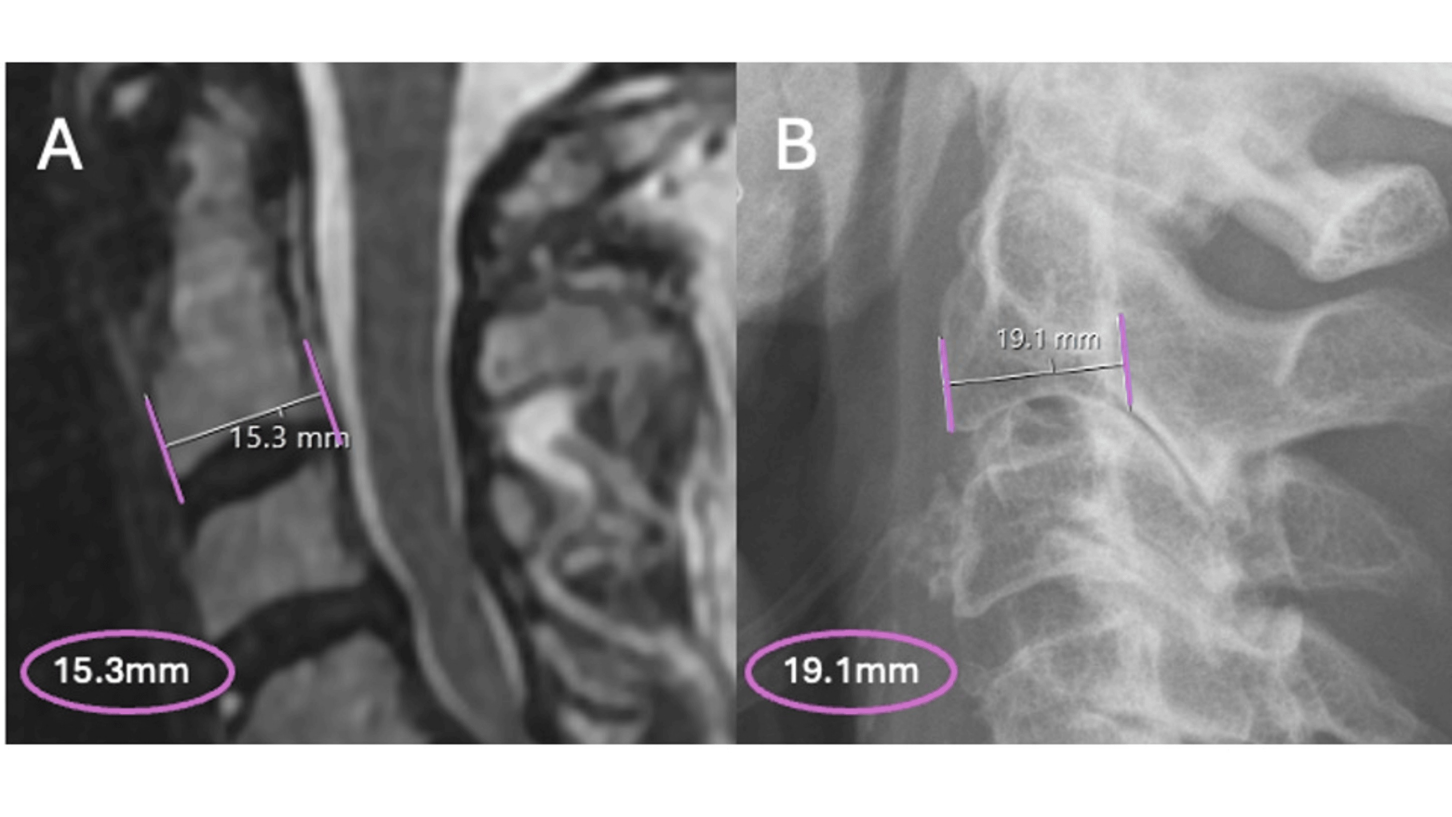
Anteroposterior measurement of the second cervical vertebrae on MRI versus X-ray of the same patient to demonstrate calibration difference A. MRI showing AP C2 distance as 15.3 mm B. Lateral cervical X-ray of the same patient showing AP C2 measurement as 19.1 mm. This highlights the importance of calculating the calibration difference to prevent errors in measurements. MRI: magnetic resonance imaging; AP: anteroposterior.
